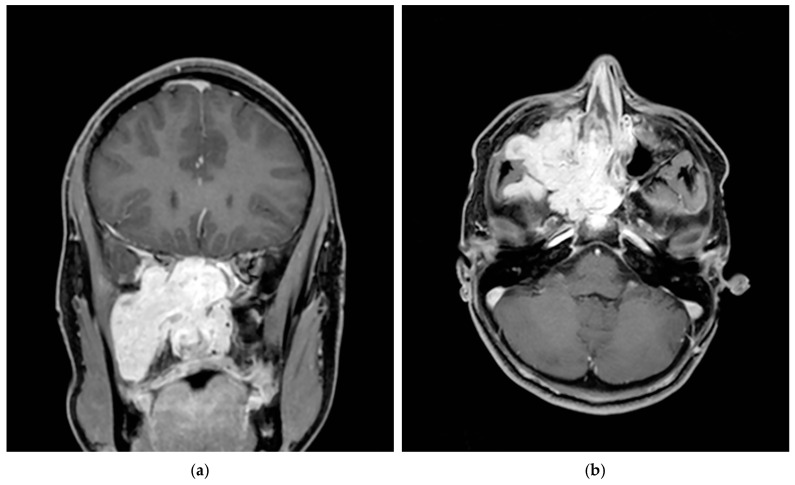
Preprocedural MRI scan (Gd-enhanced T1 Gradient-Echo 3D): coronal (a) and axial (b) views showing large JNA of right nasopharyngeal mass with expansion of the pterygopalatine fossa and extension into the infratemporal fossa.
